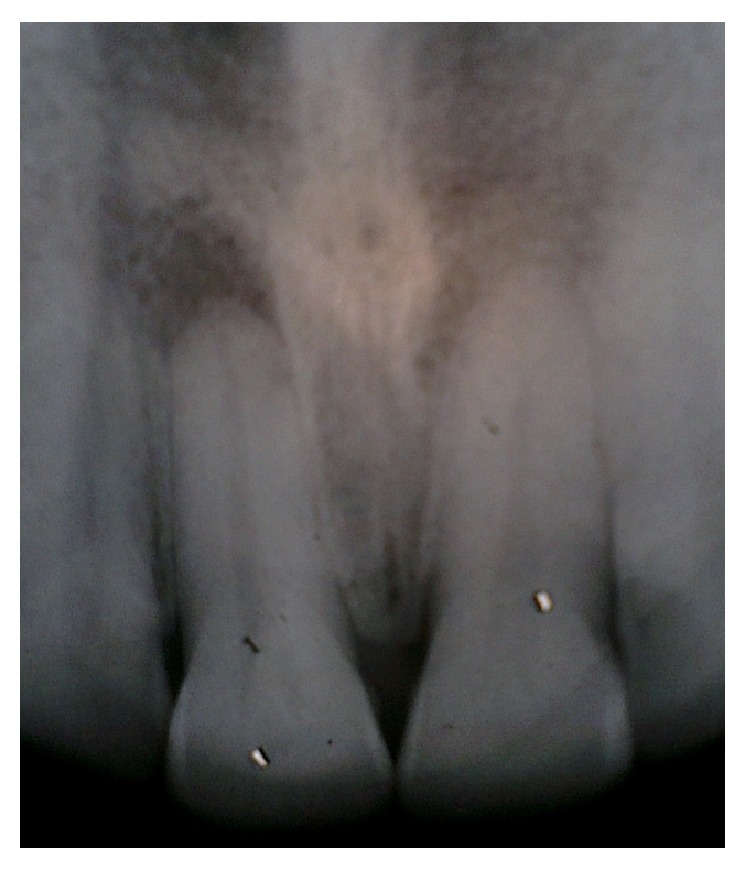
Intraoral periapical radiograph showing the periapical lesion associated with the right maxillary central incisor.
